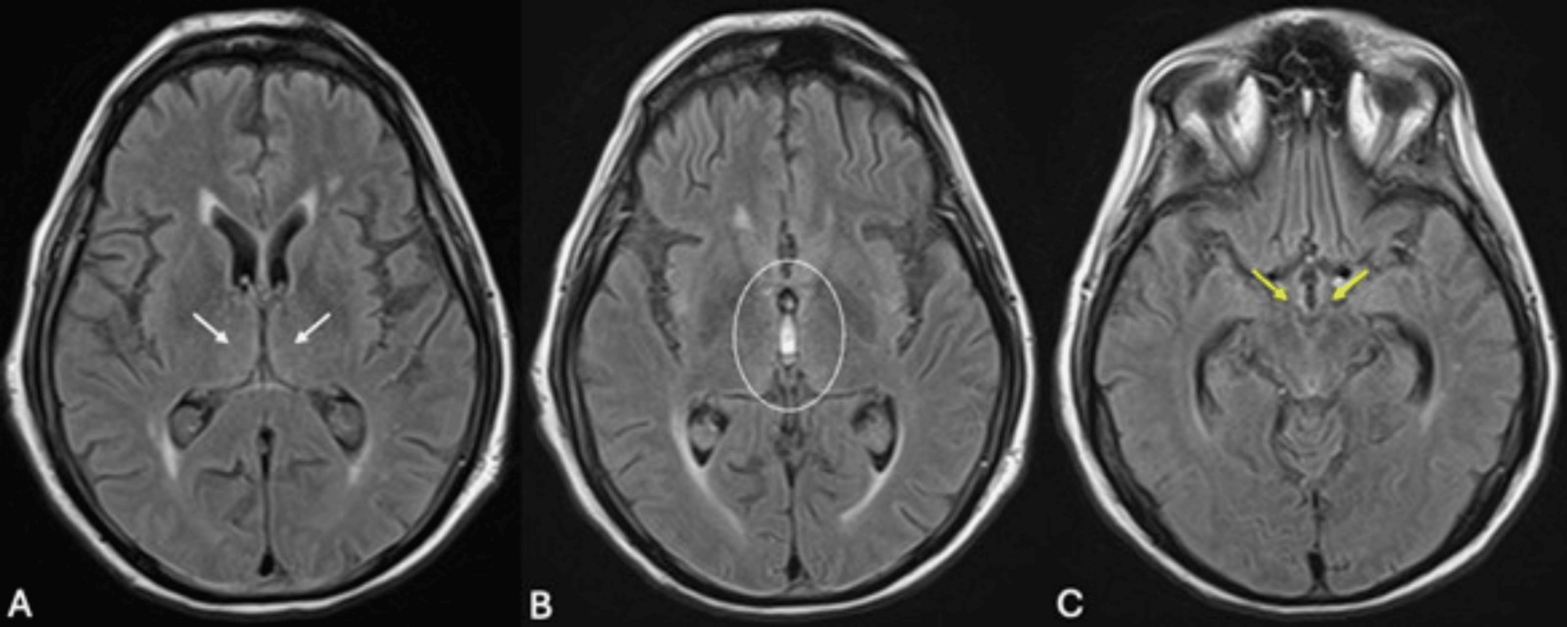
Fluid-attenuated inversion recovery (FLAIR) brain magnetic resonance (MR) images at the level of the (a) thalami (white arrows), (b) third ventricle (circle), and (c) mammillary bodies (yellow arrows) showing a normal MR signal in these regions. These areas may show increased FLAIR signal in cases of Wernicke’s encephalopathy or may be normal, as in this case.
